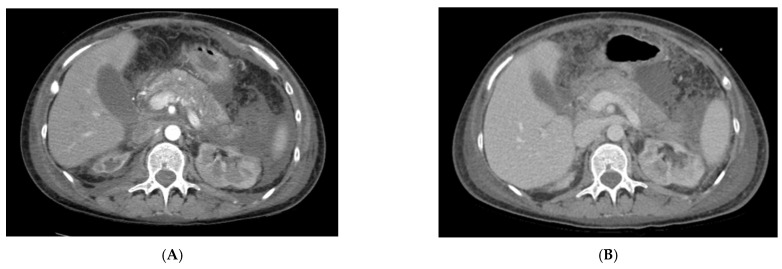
Abdominal CT scan in evolution. (A) Initial contrast-enhanced CT scan showing diffuse pancreatic edema and peripancreatic liquid collection. (B) Native CT scan revealing inhomogeneous pancreas characteristic of necrotizing pancreatitis, peripancreatic and retroperitoneal collection, and moderate ascites.
